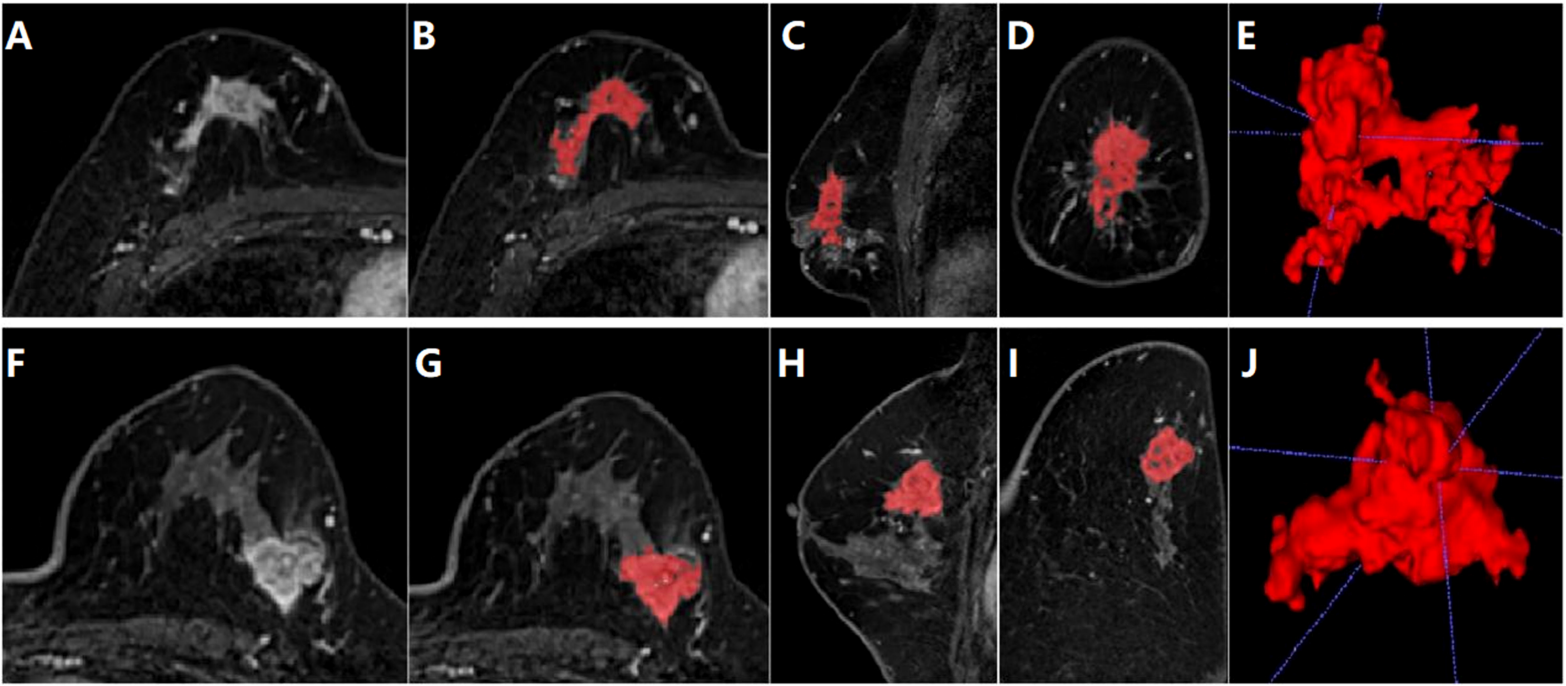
Segmentation of tumor area on DCE-MRI images of a 28-year-old female patient diagnosed with luminal type breast cancer (A-E) and a 52-year-old female patient diagnosed with non-luminal type breast cancer (F-J). (A, F) The largest slice of the lesion in axial T1WI fat-suppressed images of initial 90-seconds phase. (B, G) Delineated and then segmented lesion ROI. (C, H) Segmented lesion ROI in coronal T1WI fat-suppressed images. (D, I) Segmented lesion ROI in sagittal T1WI fat-suppressed images. (E, J) 3D view of the segmented lesion ROI.
